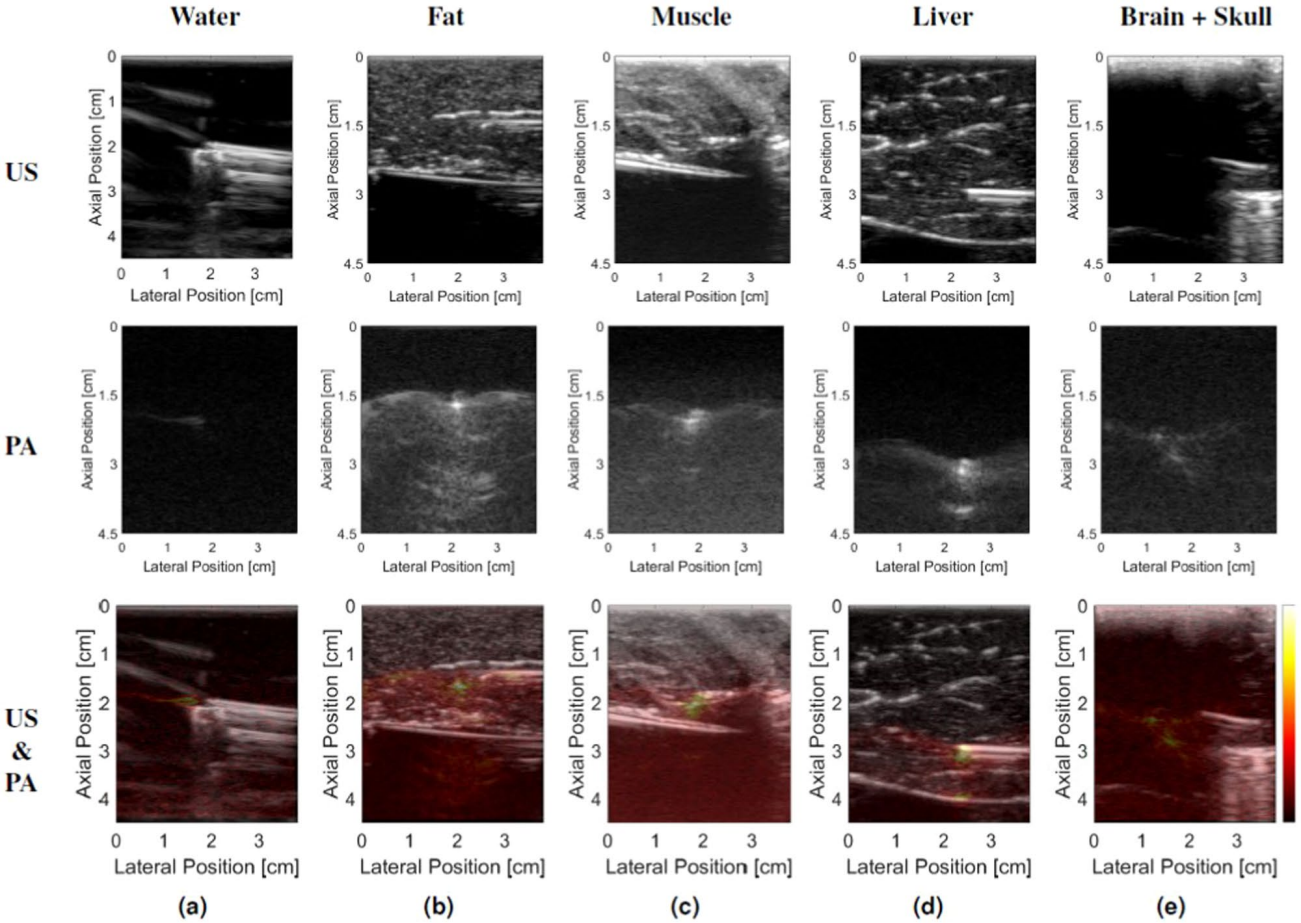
Representative ultrasound (US) and photoacoustic (PA) image pairs when visualizing the needle tip in (a) water, (b) fat, (c) muscle, (d) liver, and (e) brain tissue. Note that the brain tissue and inserted needle are not visible in the ultrasound image in the presence of 1 mm-thick cranial bone, yet we can detect the needle tip in the corresponding photoacoustic image. All photoacoustic images are shown with 60 dB dynamic range, with the color bar indicating the relative mapping used to represent the photoacoustic signal amplitudes in the overlaid photoacoustic images. All needle insertions angles were 0° relative to the lateral dimension of the ultrasound probe.
